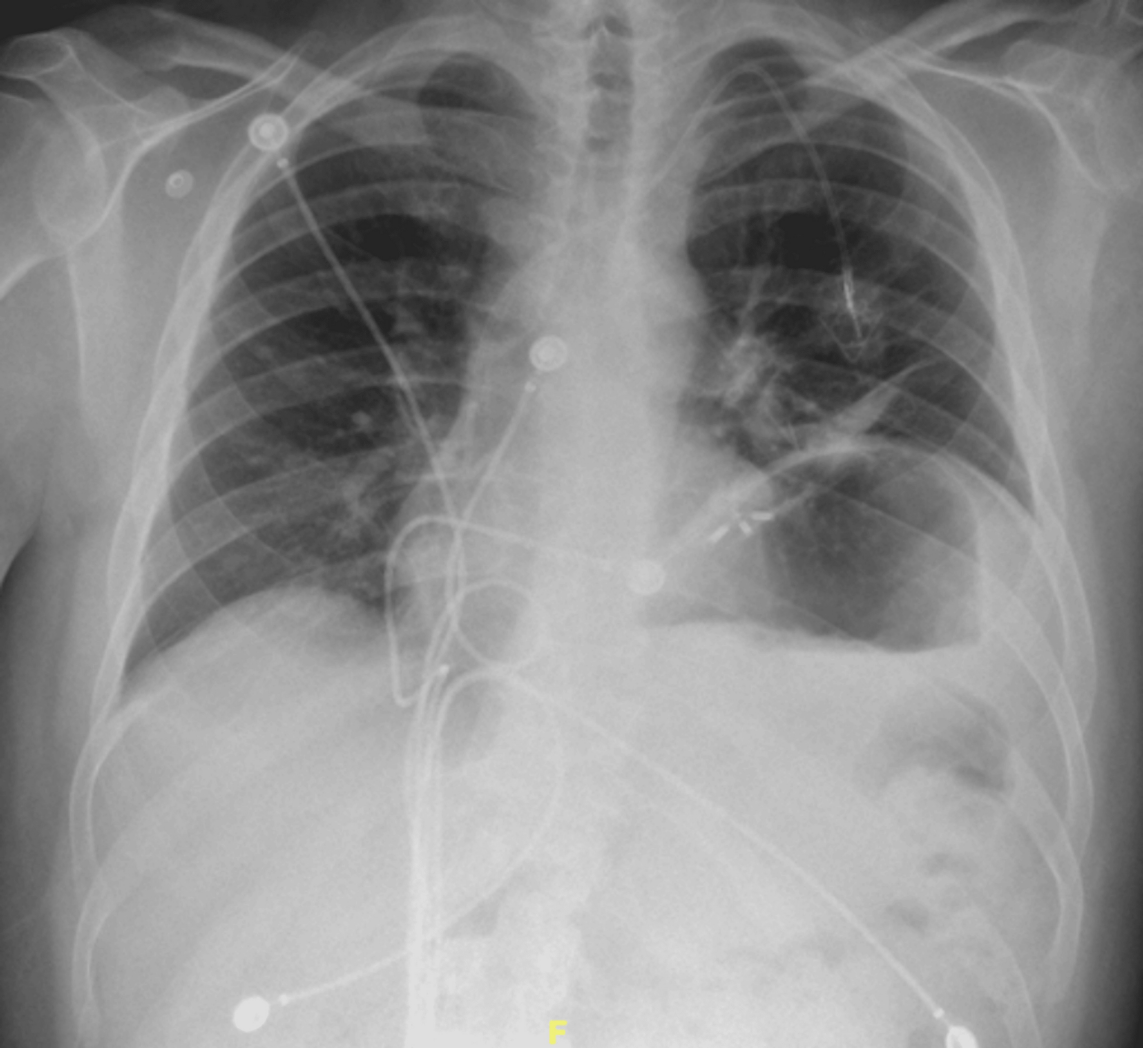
Chest radiograph reveals subsegmental atelectasis in the left lower lung zone and elevation of the left hemidiaphragm.
